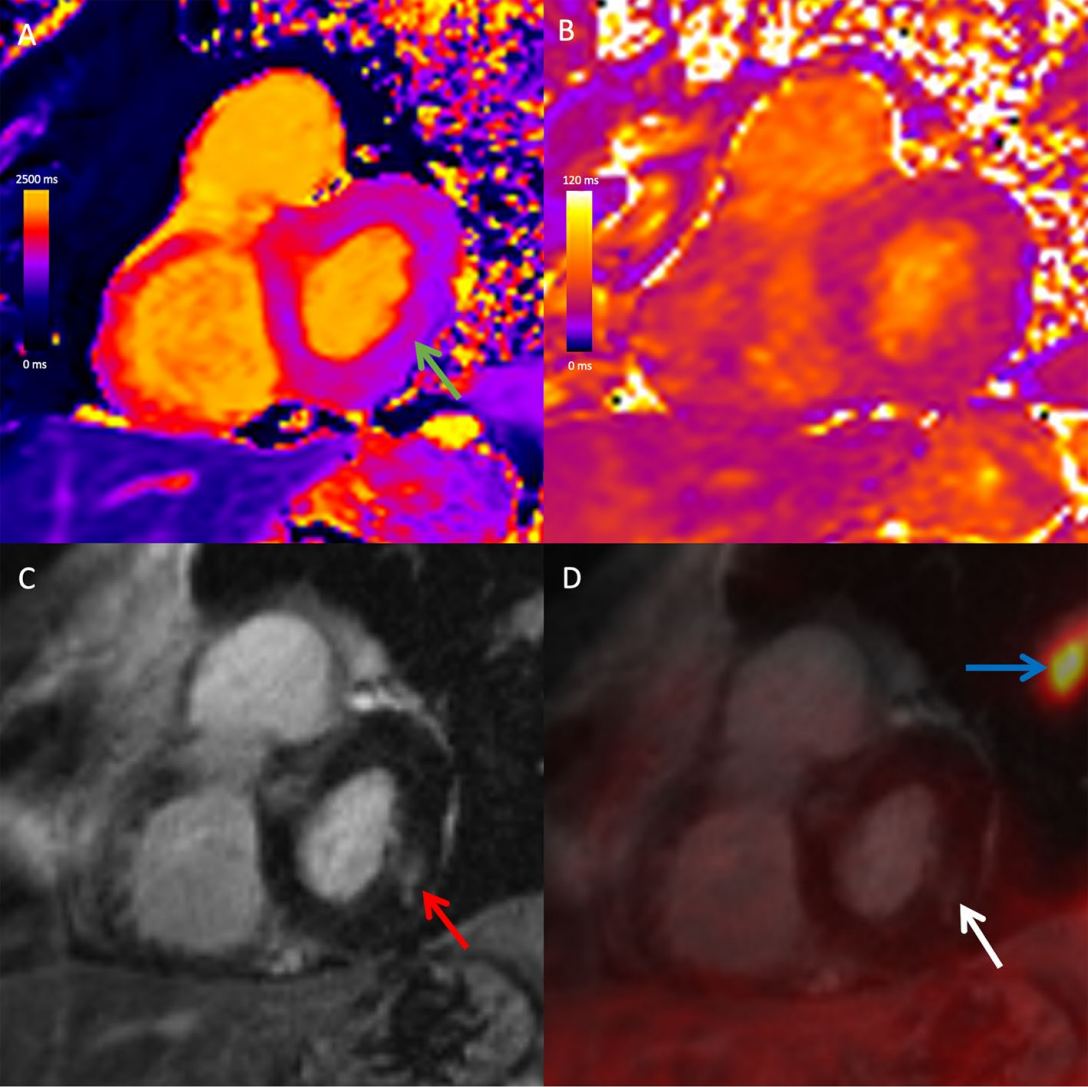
Combined 18F-FDG PET/MRI images in a 72-year-old male with cardiac and extra-cardiac sarcoidosis. Basal short-axis native T1 map (A), native T2 map (B), late gadolinium-enhanced (LGE) image (C), and fused 18F-FDG PET and LGE image (D) demonstrate slightly elevated native T1 (green arrow) and corresponding mid-wall LGE (red arrow) at the basal inferolateral wall. There was no corresponding elevation of native T2 or focal FDG uptake (white arrow). There was extra-cardiac FDG uptake in mediastinal and hilar lymph nodes (not shown) and in the lung parenchyma (blue arrow) in keeping with extra-cardiac sarcoidosis. With respect to the heart, the study was negative on PET and positive on MRI, likely reflecting chronic, burnt-out cardiac sarcoidosis
